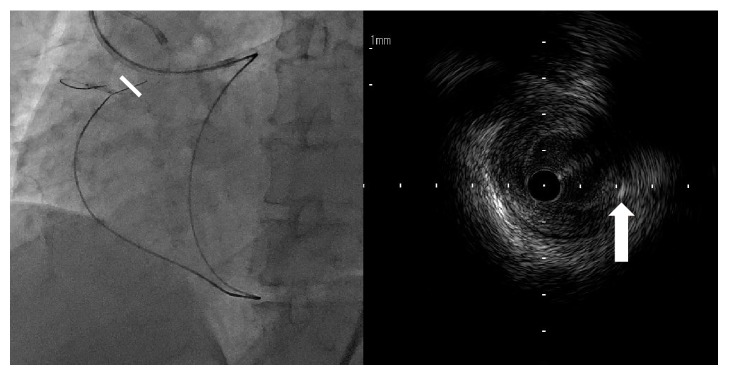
The intravascular ultrasound revealed that the retrograde guidewire (white arrow) was in the subintimal space at the proximal right coronary artery past the chronic total occlusion.
